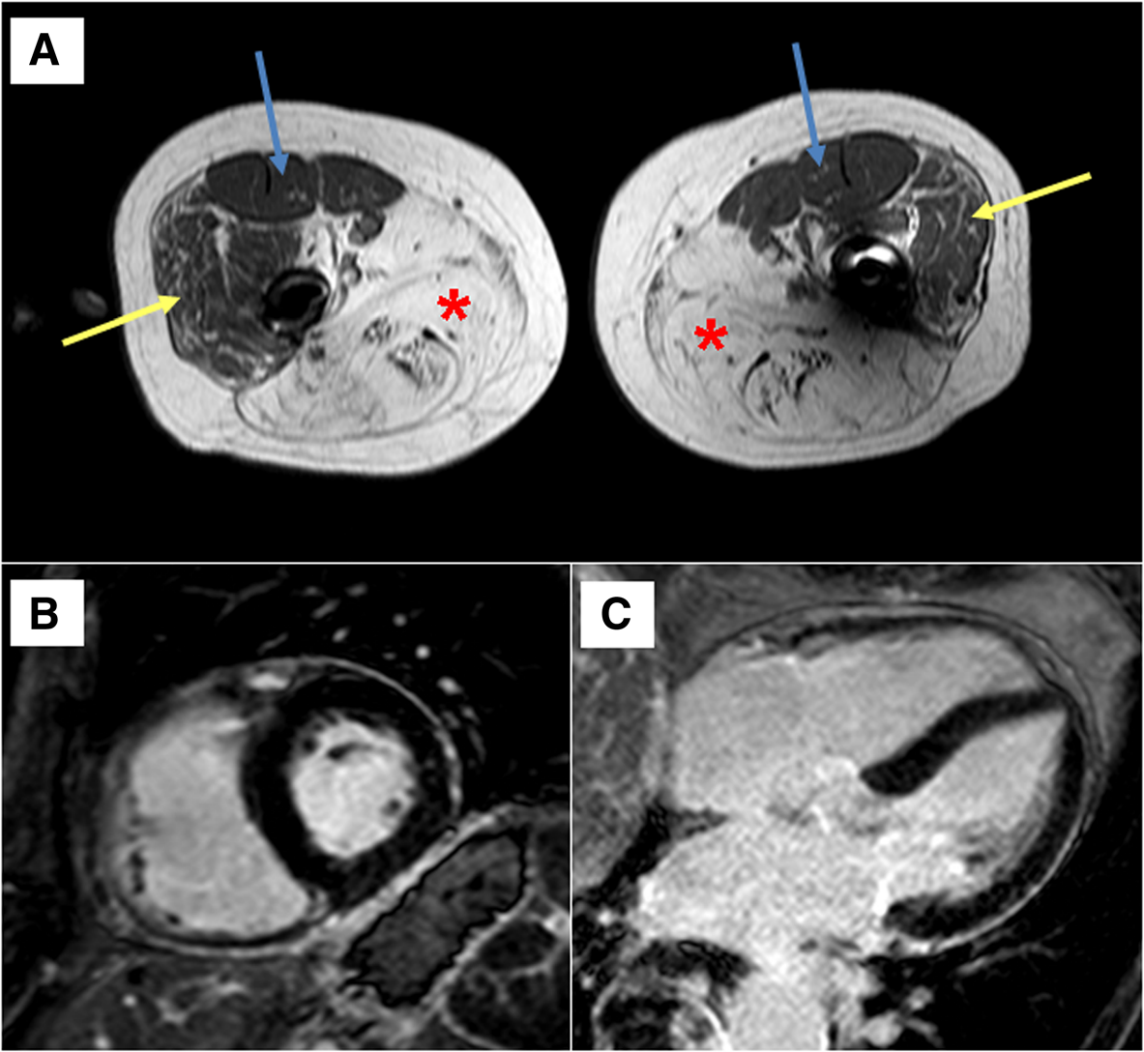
a Transverse T1-weighted spin-echo CMR of the thigh musculature in a patient with late-onset Pompe disease (LOPD). The adductor and ischiocrural muscles show symmetrical and confluent hyperintensity indicative of severe fibrosis and lipodystrophy (*red stars*). Some patchy fatty replacement can also be seen in the vastus lateralis muscles (*yellow arrows*) and, to a much lesser extent, in the vastus medialis muscles (*blue arrows*). b–c Short and long-axis late gadolinium enhancement (LGE) CMR images of the same patient showing no myocardial involvement (no fibrosis, no fatty replacement)
